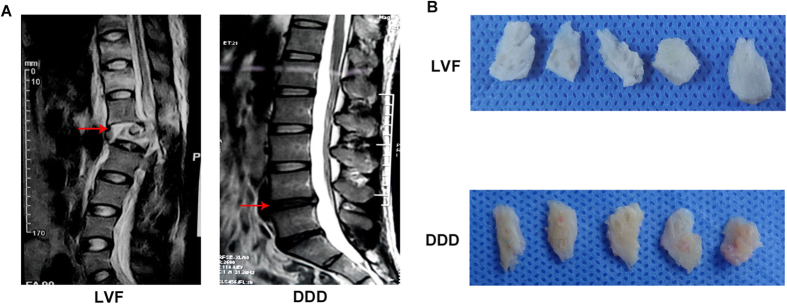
Representative MRIs and CEP samples. (A) MRI scans of spines with LVF and DDD. Featured left is a patient with lumbar LVF undergoing posterior discectomy, spinal fusion, decompression, and stability within 24 hours of trauma. This patient had no formerly documented clinical history of low back pain (LBP). Featured right is a patient with DDD undergoing discectomy and intervertebral fusion surgery. The red arrow indicates the position of the experimental material. (B) CEP samples carefully isolated from IVD tissue with a scalpel.
